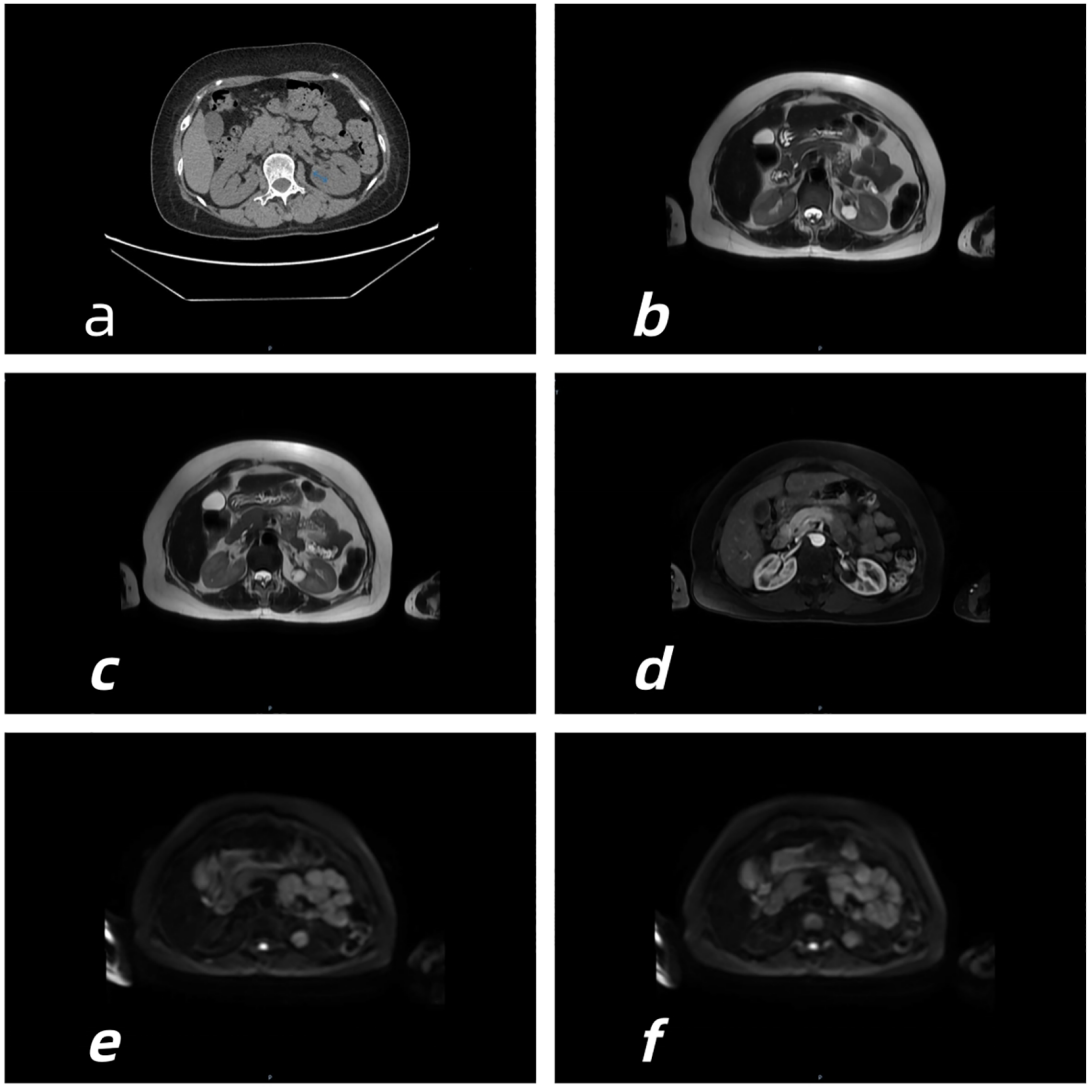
CT and MRI imaging of RCCC.
